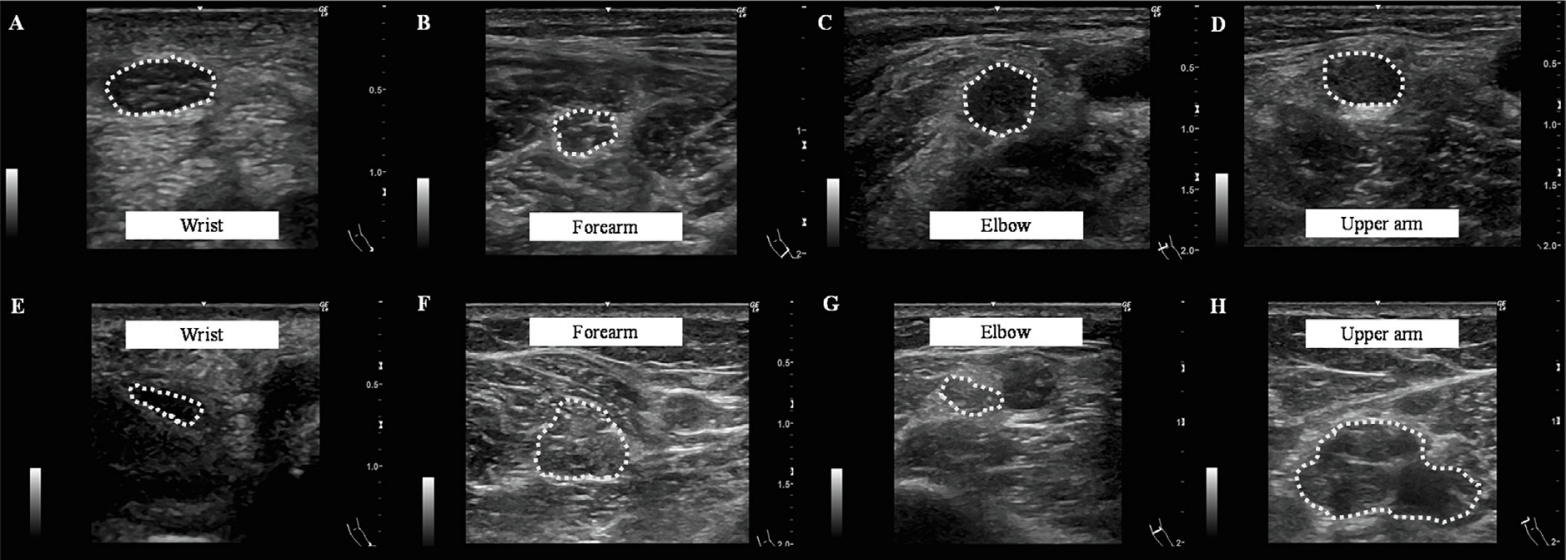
Representative ultrasound findings of the median nerve in a 65-year-old man with typical chronic inflammatory demyelinating polyneuropathy (CIDP) (A–D) and in a 53-year-old woman with multifocal CIDP (E–H). Each image shows the cross-sectional area (CSA) at the wrist (A = 18 mm^2^, E = 9 mm^2^), forearm (B = 16 mm^2^, F = 33 mm^2^), elbow (C = 26 mm^2^, G = 11 mm^2^), and upper arm (D = 21 mm^2^, H = 55 mm^2^). The wrist-to-forearm index (WFI) is 1.13 (typical CIDP) vs. 0.27 (multifocal CIDP); elbow-to-upper arm index (EUI) is 1.24 (typical CIDP) vs. 0.20 (multifocal CIDP); intranerve CSA variability (INCV) is 1.63 (typical CIDP) vs. 6.11 (multifocal CIDP).
